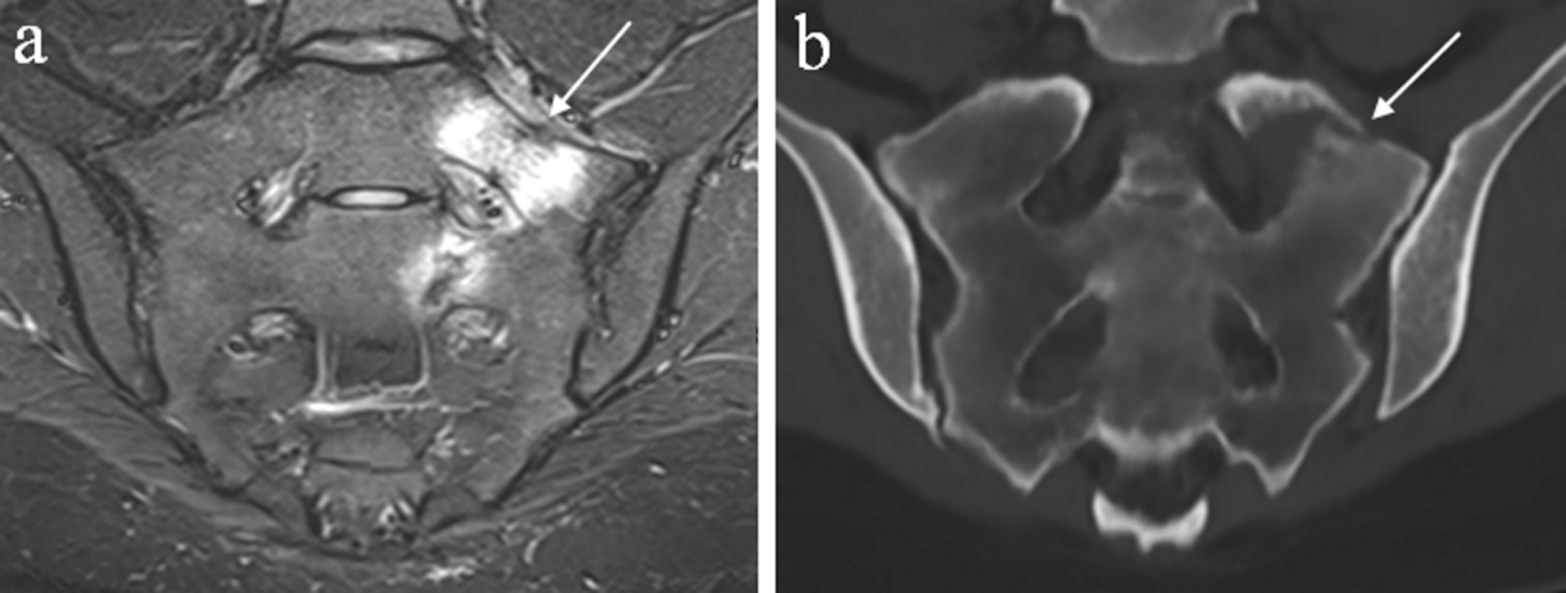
Synthetic CT and the T2 Short TI Inversion Recovery (STIR) sequence are complementary for diagnosis. A 22-year-old female with low back pain irradiating towards the left calf shows a left sacral stress fracture with bone marrow edema on the semicoronal STIR image (a) and associated cortical disruption on semi-coronal synthetic CT (b).
